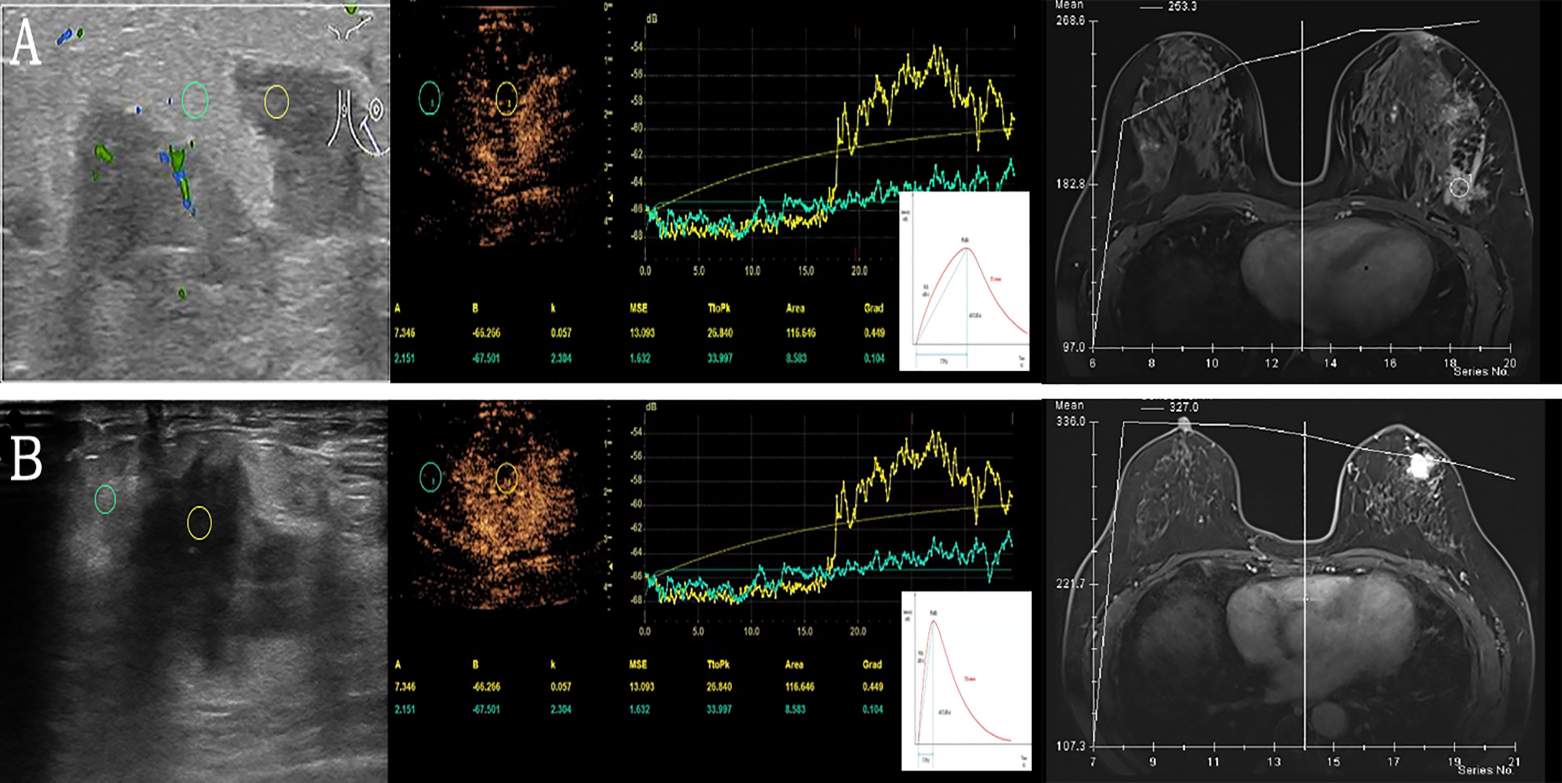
Examples of quantitative data acquisition using ROI. (A) Granulomatous lobular mastitis in a 28-year-old female. Moderate enhancement of the lesion (yellow ROI) compared to minimal enhancement in surrounding breast tissue (green ROI). Gradual enhancement and a gradual wash out of contrast agent (corresponding TIC below) versus TIC of DEC-MRI. (B) Invasive ductal carcinoma in a 61-year-old female with significant enhancement of the lesion (yellow ROI) compared to slight enhancement in surrounding breast tissue (green ROI), the lesion is ill-defined. After rapid enhancement of the tumor, early wash-out can be observed (corresponding TIC below) versus TIC of DEC-MRI. ROI, region of interest; TIC, time intensity curves; DEC-MRI, dynamic contrast enhancement magnetic resonance imaging.
